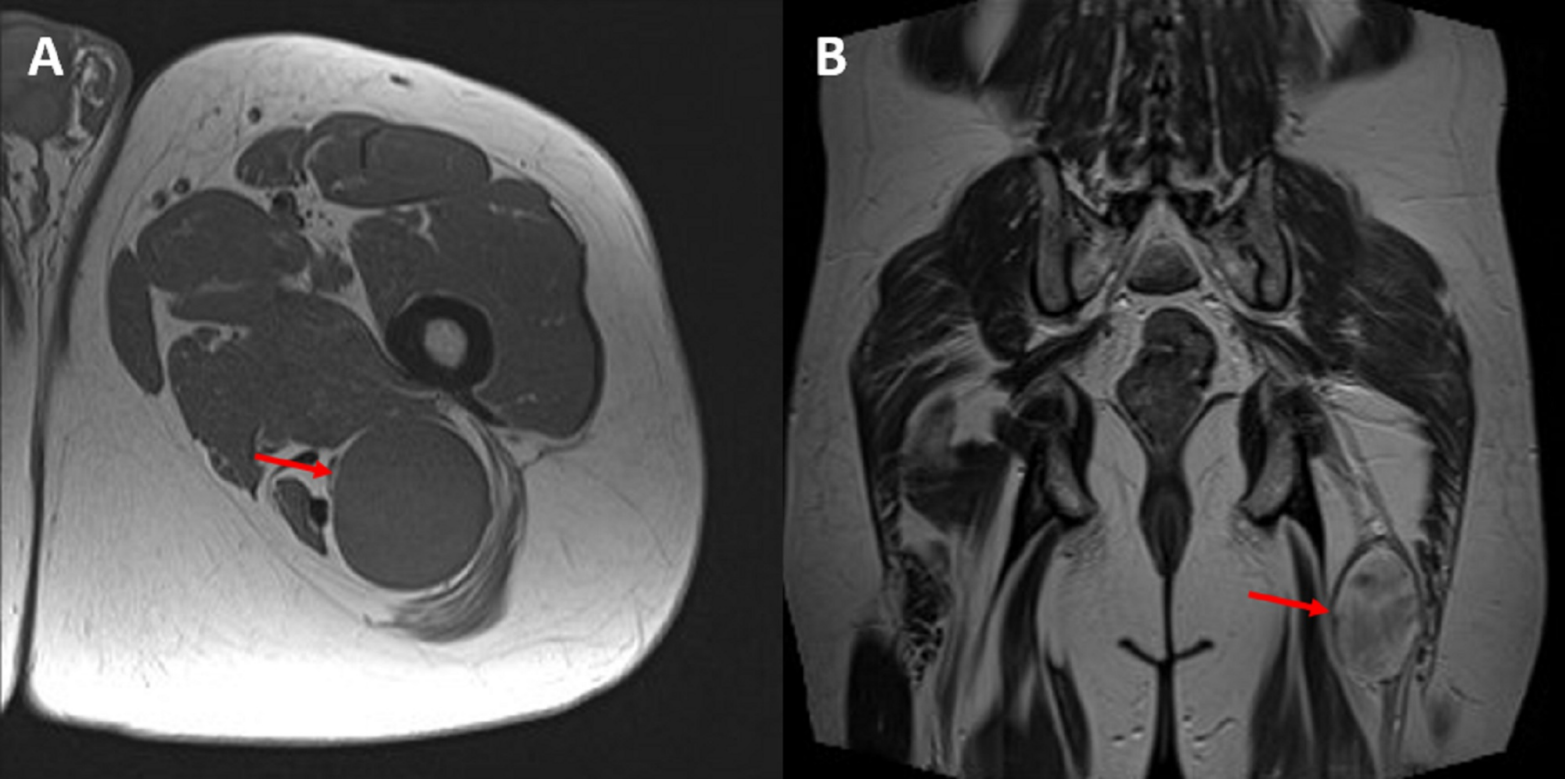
Imaging of sciatic tumor 2A. Axial T1 and 2B. Coronal T2-weighted MR images demonstrating the sciatic nerve tumor (arrow) in the posterior left thigh that was resected uneventfully shortly after the initial presentation with a resolution of the patient’s long-standing left sciatica MR: magnetic resonance
